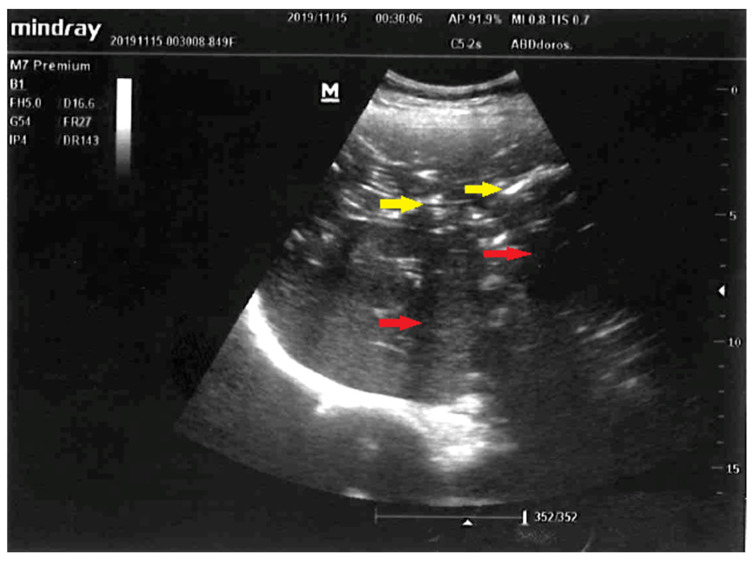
Early liver ultrasound (US) performed to find a source of sepsis in a 52-year-old female suspected to have developed intraabdominal sepsis. Visualized here are: normal-sized liver, punctuated hyperechoic foci with anti-gravitational distribution (yellow arrows) casting acoustic shadows (red arrows). Due to characteristic appearance on liver US, the most likely diagnosis was hepatic portal venous gas (HPVG) [1,2]. Other less likely diagnoses were: pneumobilia due to cholangitis, hepatic micro-abscesses, and punctuate calcifications. Hepatic portal venous gas detected by US following liver transplantation or in suppurative cholangitis were described previously [3,4]. Although presence of mesenteric ischemia and gas in the portal venous circulation was previously revealed with computed tomography [5,6], in our case early abdominal US was performed, what expedited definite diagnosis and targeted treatment: choice of empiric broad-spectrum antibiotics and explorative laparotomy. This is the first time, to the best of our knowledge, that HPVG was visualized with US in the setting of acute mesenteric ischemia.
